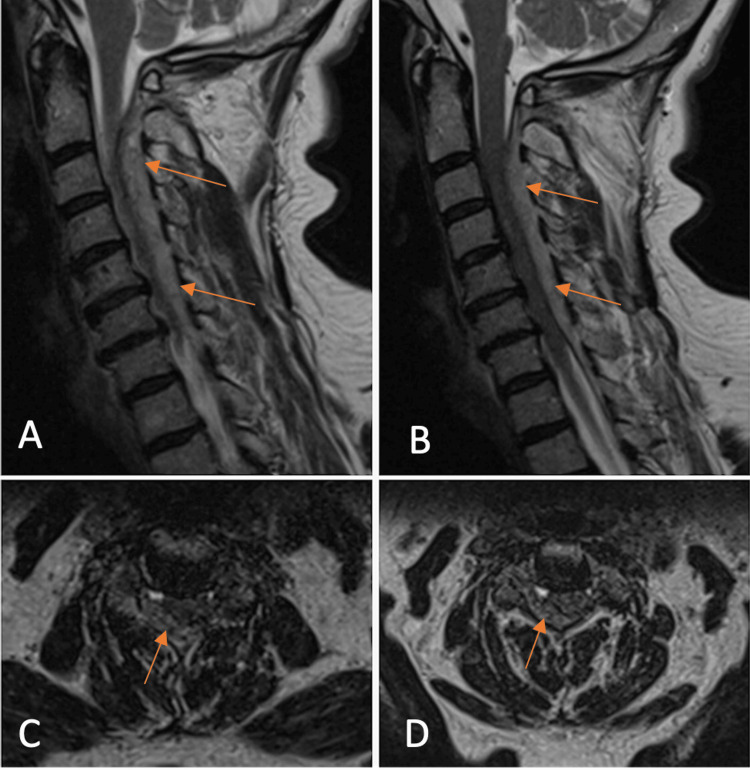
T2-weighted magnetic resonance imaging (MRI) of the second case demonstrating a spontaneous spinal epidural hematoma (SSEH) (arrows) on the right posterolateral side extending from C2 to C7. (A) Sagittal image paramedian on the right side; (B) midline sagittal image; (C) axial image at C3; (D) axial image at C5.
